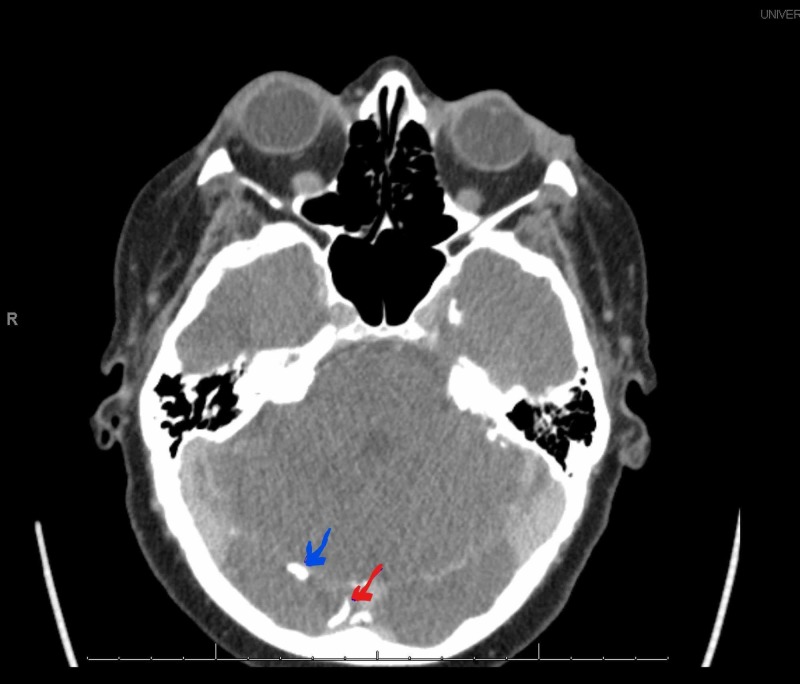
CT head showing calcifications along the falx and tentorium cerebelli. The blue arrow is showing calcifications along the tentorium cerebelli, and the red arrow is showing calcifications along the falx cerebelli.
